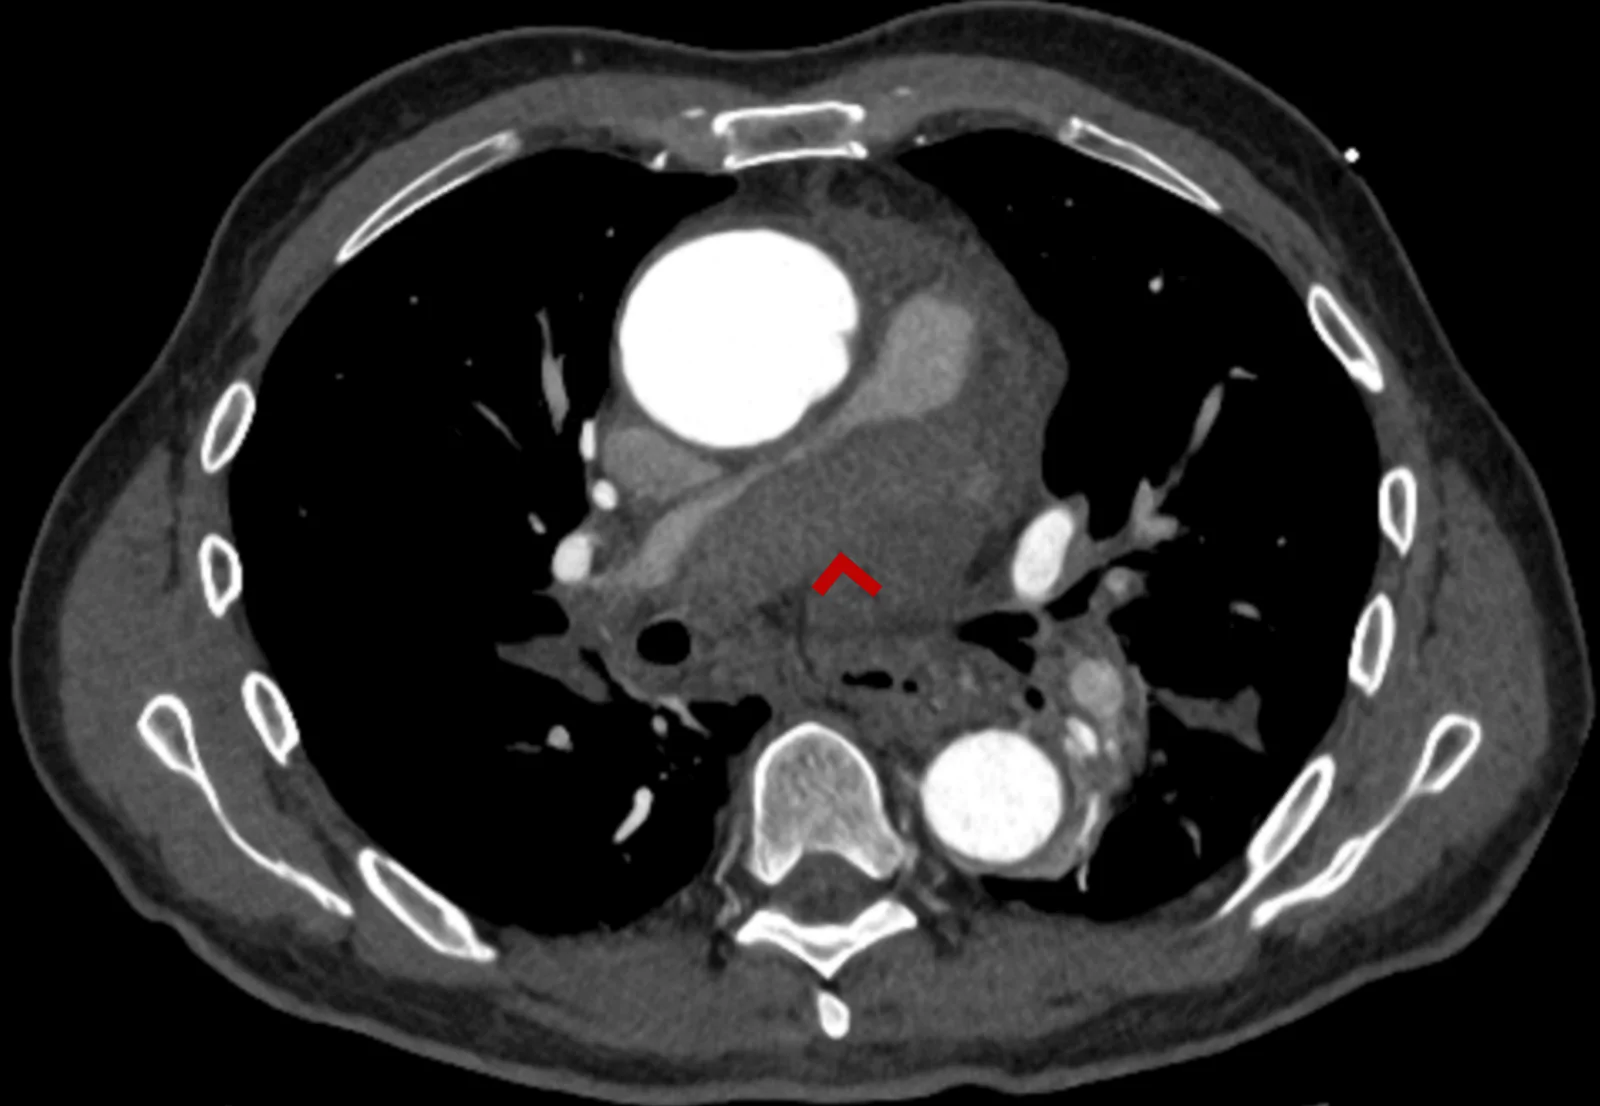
CT scan, axial arterial phase imaging. The red arrow indicates haematoma within the sheath of the great vessels partially effacing the pulmonary trunk.
